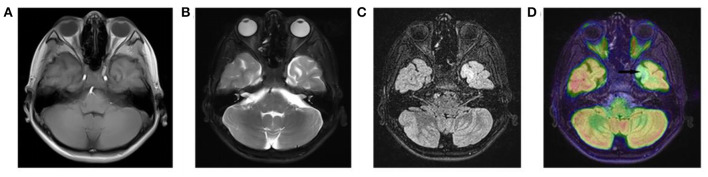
The Cranial MRI and PET-MR image fusion of case 1. There are (A) mixed T1WI signals, (B) long T2WI signals and (C) long T2-FLAIR signals in the left temporal lobe. (D) PET-MR image fusion shows decreased metabolism (arrow) in the corresponding positions.
